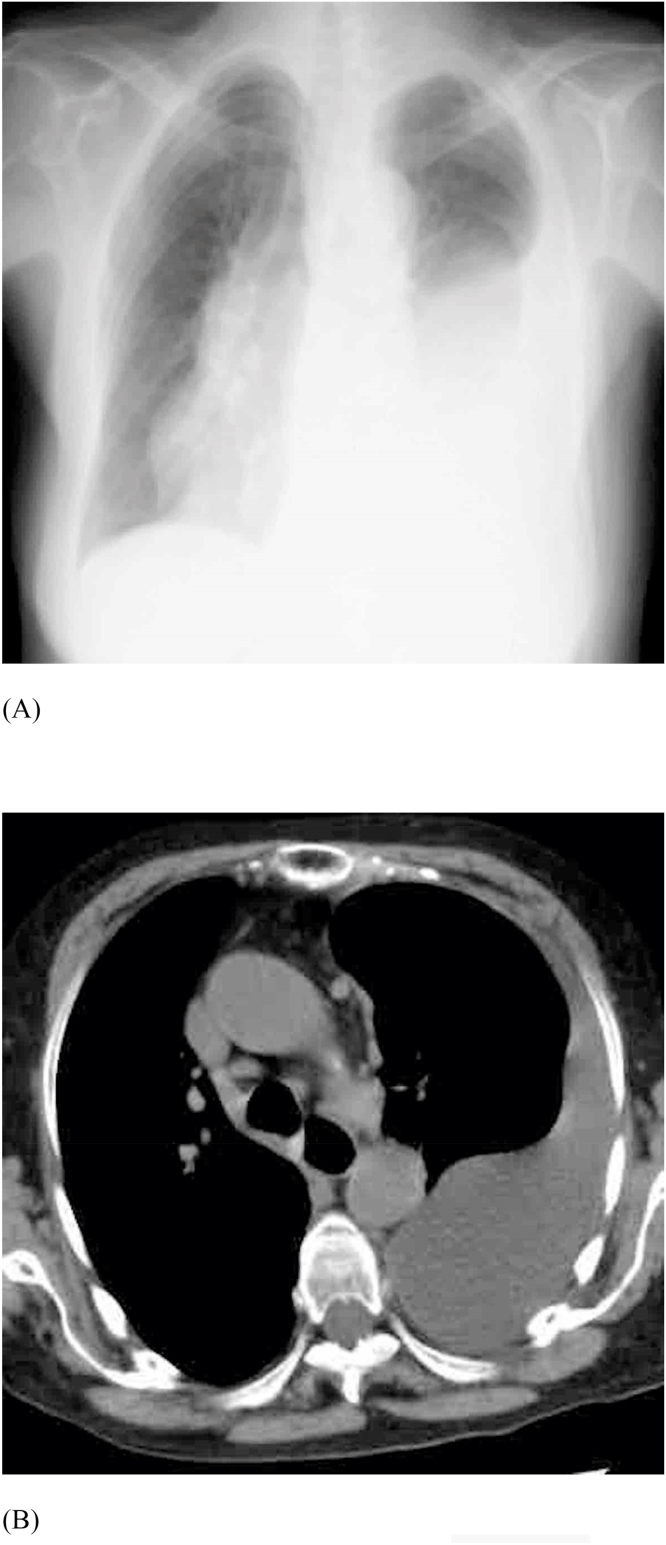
Chest radiogram (A) and computed tomography image (B) show left pleural effusion and mild lymphadenopathy. No obvious infiltrative shadows or interstitial shadows are seen in the lung field.
